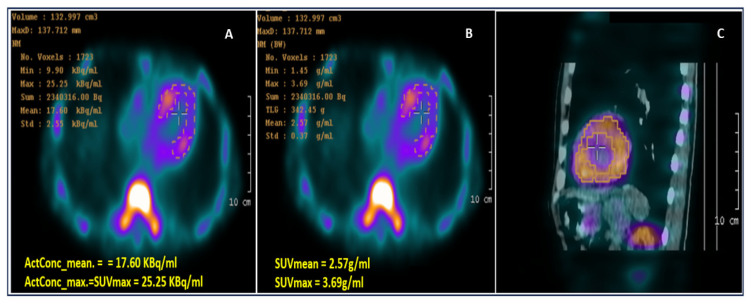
Computed 99mTc-PYP SPECT/CT images: (A) Activity concentration (ActCon) image; (B) SUV image; and (C) fused SPECT-CT image showing myocardium segmentation based on the thresholding method (40% of peak activity) as suggested by Caobelli F. et al. [9]. CT: computed tomography; SPECT: single-photon emission computed tomography; SUV: standardized uptake value; 99mTc-PYP: 99mTechnetium-pyrophosphate.
